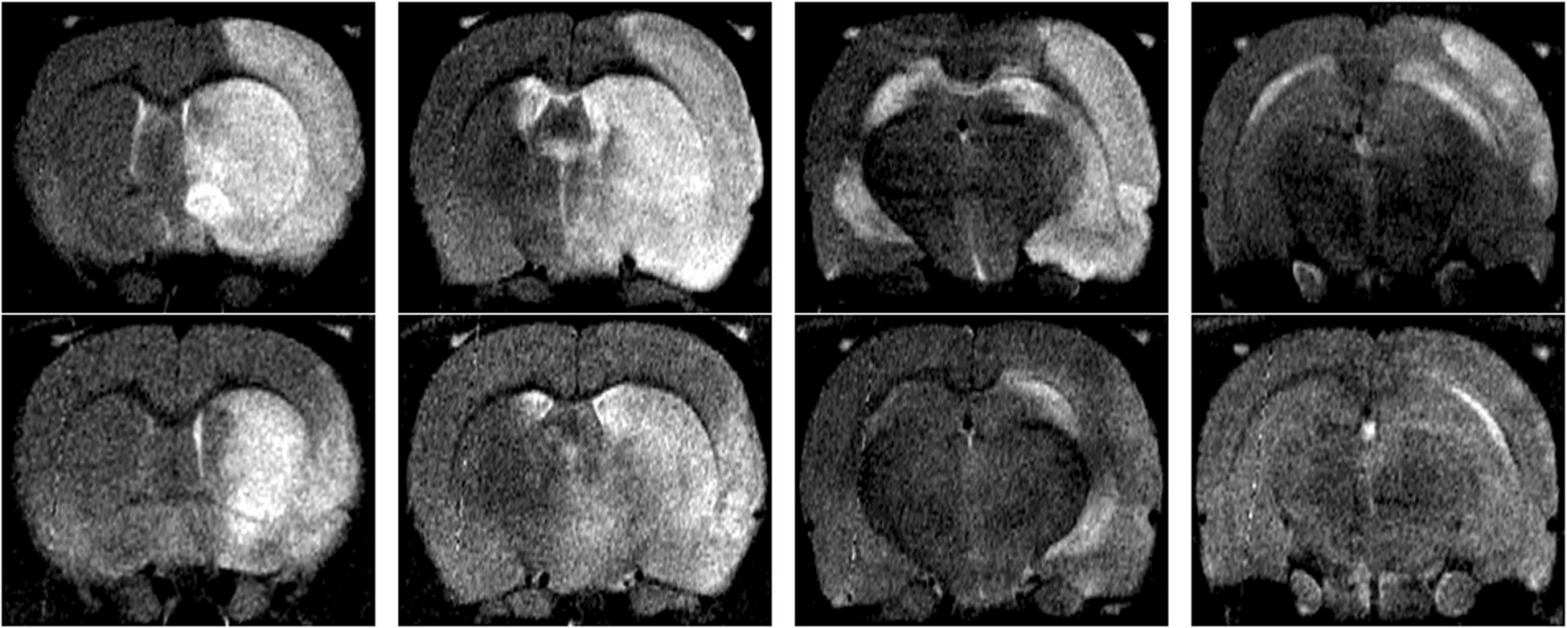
T2-weighted MRI. Representative examples of right-hemispherical ischemia in T2-weighted MRI after 24 h (contiguous slices 2–5): 1^st^ row NMO-IgG, 2^nd^ row control-IgG.
